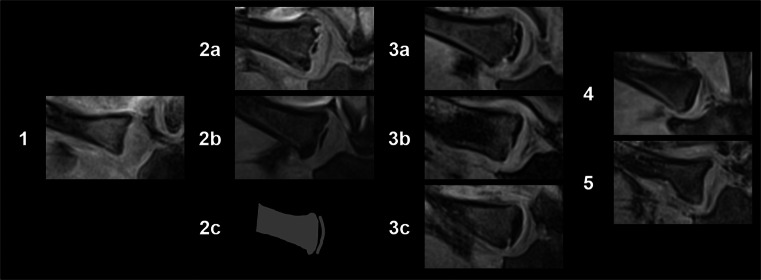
Representative MR images (T1w 3D VIBE FS) of the clavicle stages. Stage 2c was not found in the current study; therefore, a sketch is presented
